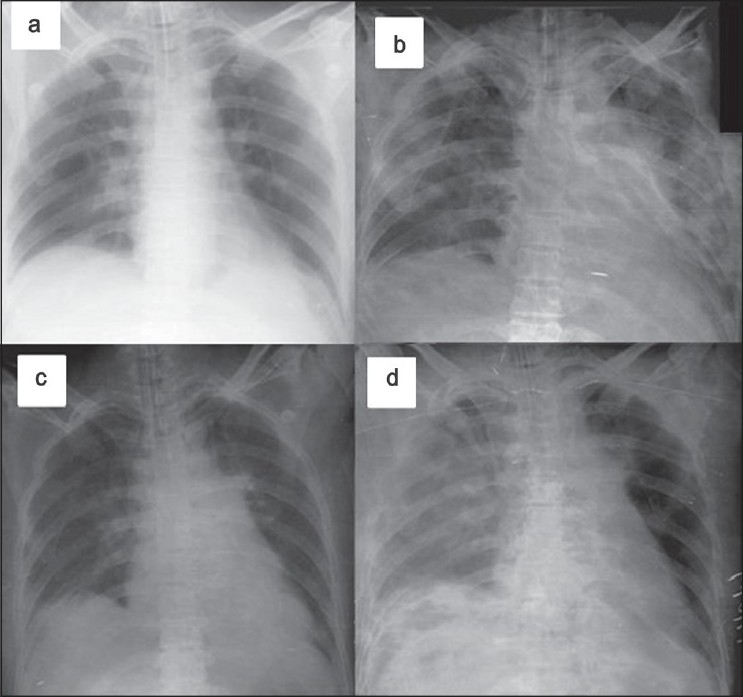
Chest radiographs of the patient. (a) Normal chest radiograph on Day 15, (b) Left lower lobe collapse and infiltration on Day 16, (c) Two hours after recruitment on Day 16, (d) Infiltration of right lung on Day 17.
